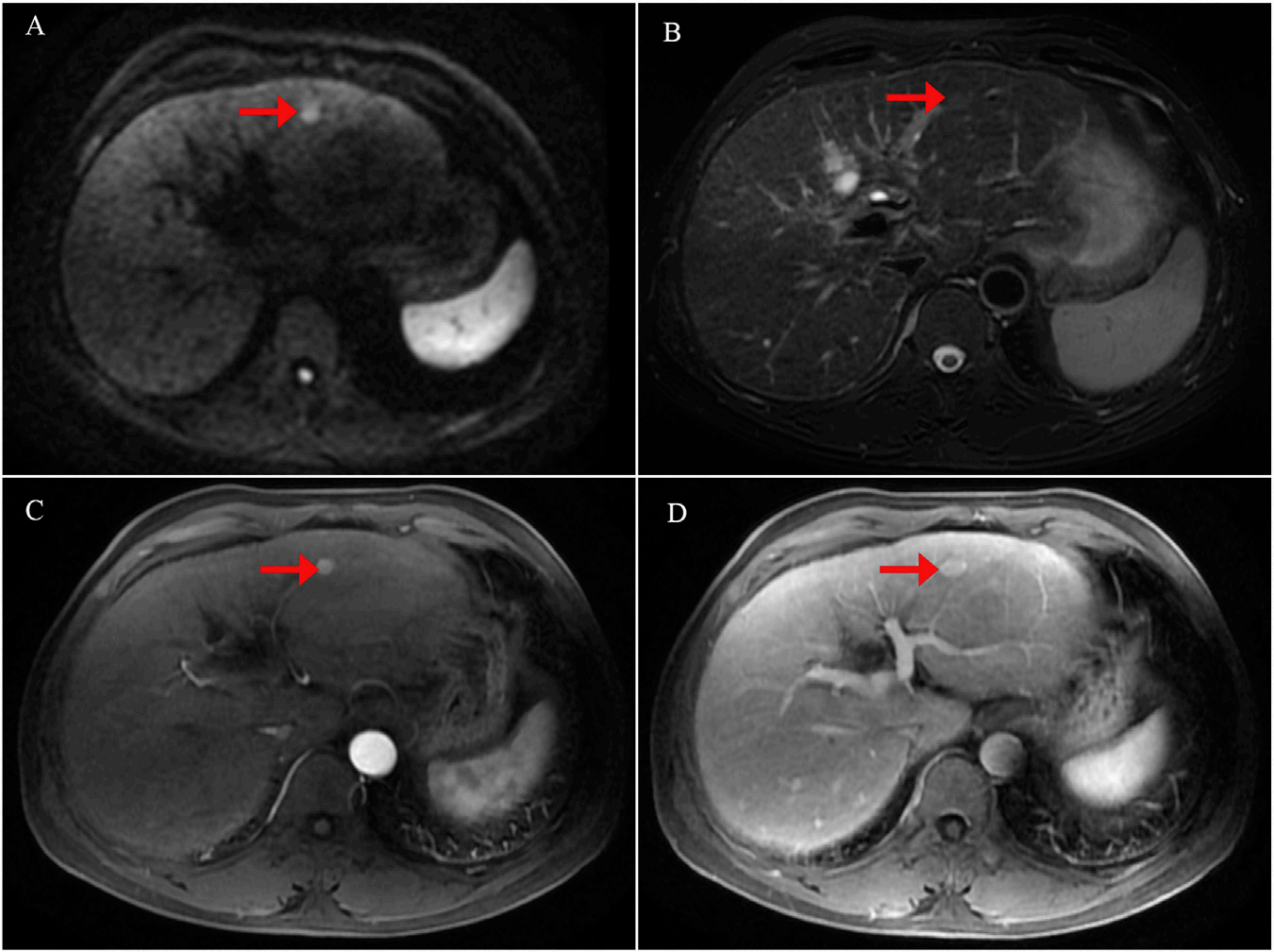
Initial contrast-enhanced liver magnetic resonance imaging (MRI) findings at the time of diagnosis. (A) Diffusion-weighted imaging (DWI) showing a focal area of restricted diffusion in the liver. (B) T2-weighted image demonstrating corresponding hyperintensity in the lesion. (C) Arterial phase of contrast-enhanced MRI revealing early enhancement of the lesion. (D) Venous phase image showing washout or delayed enhancement characteristics.
